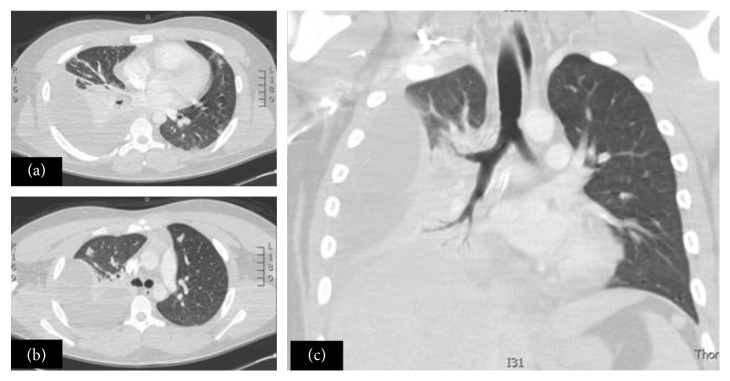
(a) and (b) CT scan chest (severe consolidation with effusion). (c) Coronal image of CT chest showing extensive consolidation and para pneumonic effusion.
